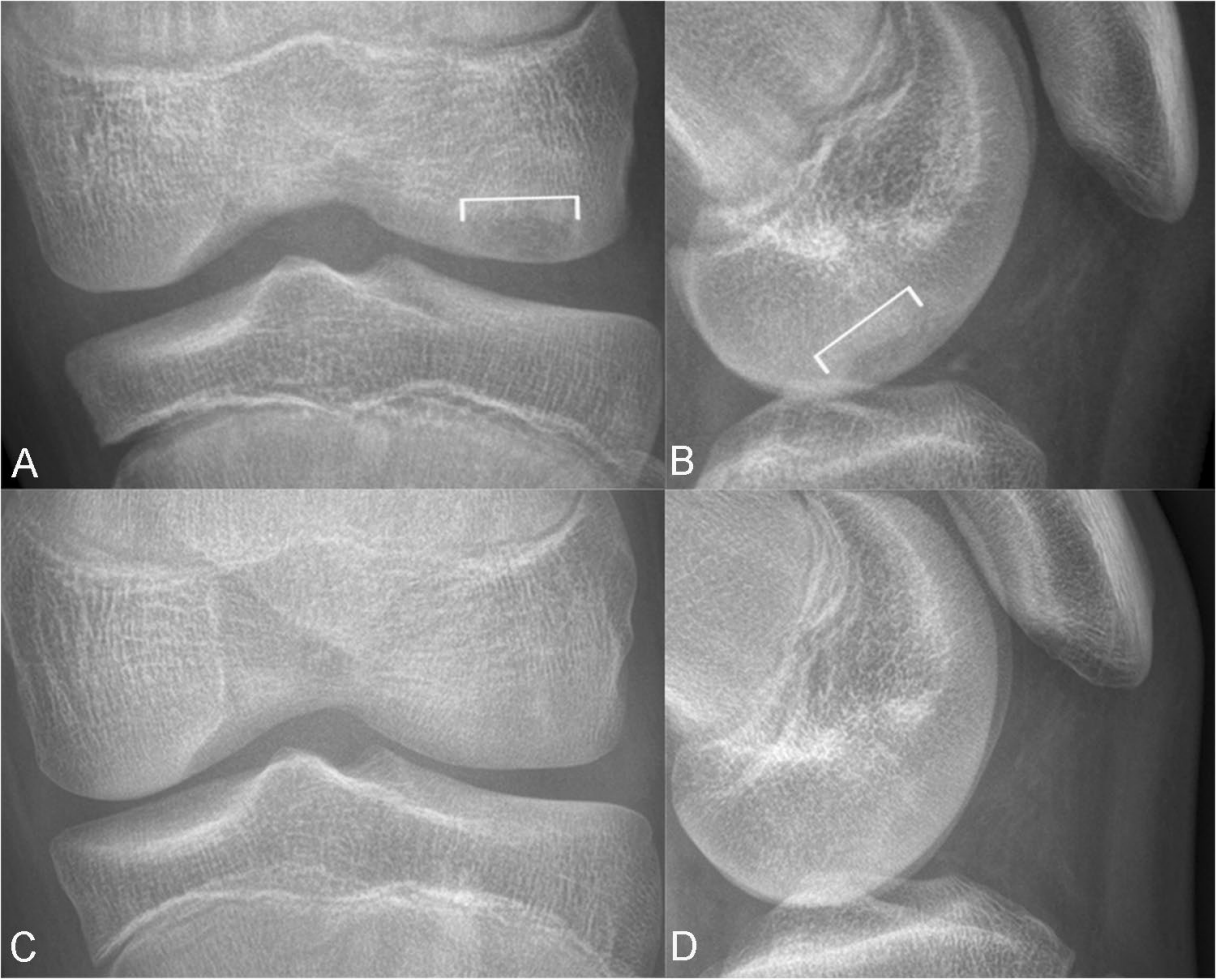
Conservatively treated LFC OCD lesion in a 13-year-old skeletally immature male. Anteroposterior (A, C) and lateral (B, D) radiographs at initial presentation (A, B) and 2 years later (C, D) show a less conspicuous progeny (brackets, A-B), no progeny-parent bone interface, or development of parent bone marginal sclerosis. Patient reported resolved symptoms.
